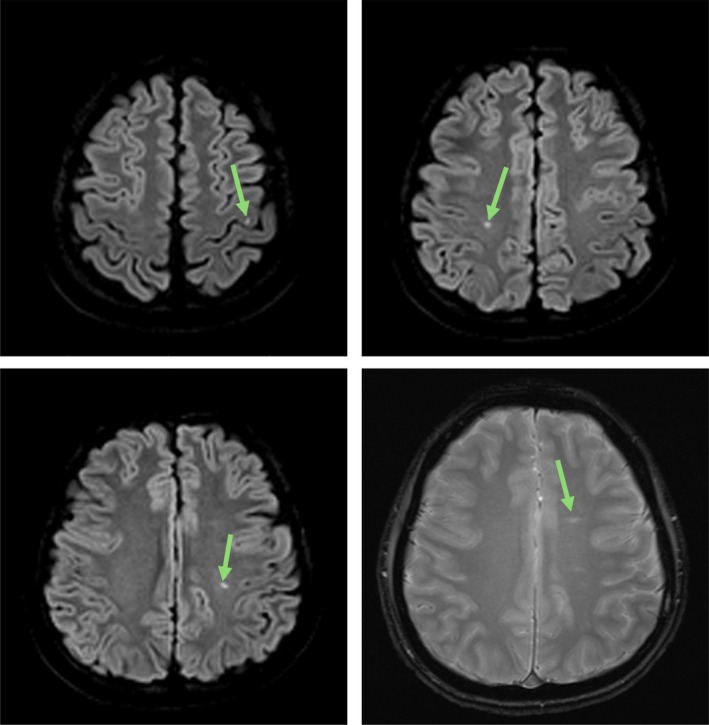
Brain MRI showing three foci of acute infarcts (top two images and bottom left, DWI sequence) and signs of early chronic microvascular ischemic changes (bottom right, T2/FLAIR sequence).
